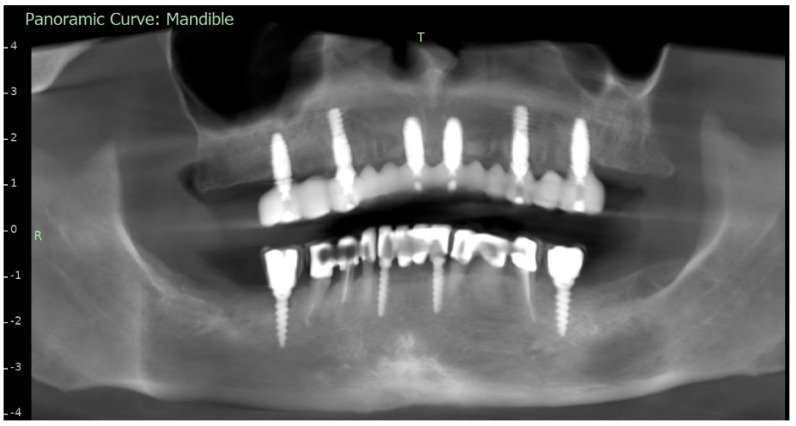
Control CBCT scan obtained after the delivery of the early full-arch provisional restoration.
